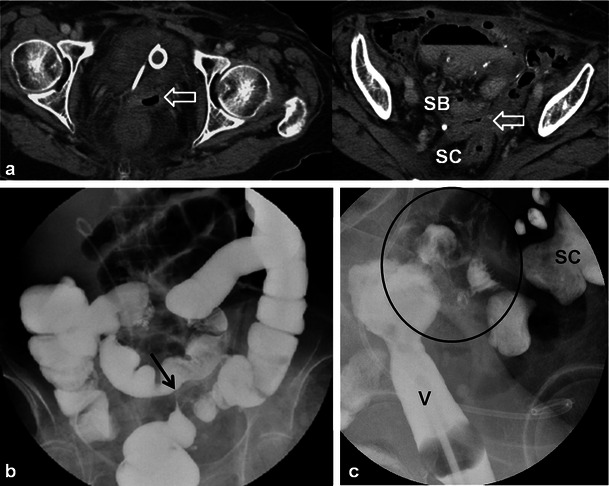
An 80-year-old woman with a history of endometrial carcinoma and ovarian carcinoma treated remotely with pelvic surgery and RT presenting with clinical symptoms of vaginal-enteric fistula. Initial CECT axial images (a) depict abnormal gas within the thickened vagina (*open white arrow*) which is in close proximity to both pelvic small bowel loops (*SB*) and sigmoid colon (*SC*). A colovesical fistula was suspected. Barium enema was subsequently performed (b) to confirm the findings. Overhead AP image from single contrast barium enema fails to demonstrate communication between the colon and vagina. Note short segment persistent narrowing of the sigmoid colon (*black arrow*) with tapered margins and preserved mucosal folds (not shown) consistent with radiation induced stricture. Direct vaginography was suggested for further evaluation. Oblique radiograph from direct vaginogram depicts maximal distention of the vagina (*V*) and residual barium in the sigmoid colon (*SC*) with uncomplicated diverticulosis. There is abnormal filling of pelvic small bowel loops (*black circle*). The findings were in keeping with fistula between the distal ileum and vagina
